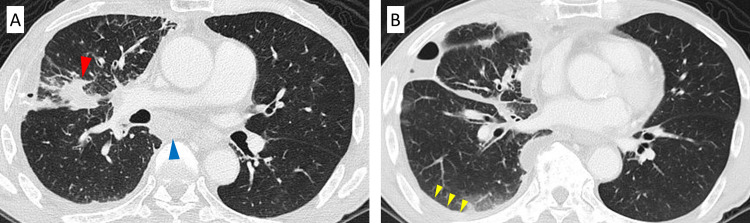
Chest computed tomography before the initiation of treatment with pembrolizumab Chest computed tomography before the initiation of treatment with pembrolizumab showed the primary tumor in right upper lung (red arrow), swelling of #7 lymph node (blue arrow) (A), and right mild pleural effusion (yellow arrows) (B).
